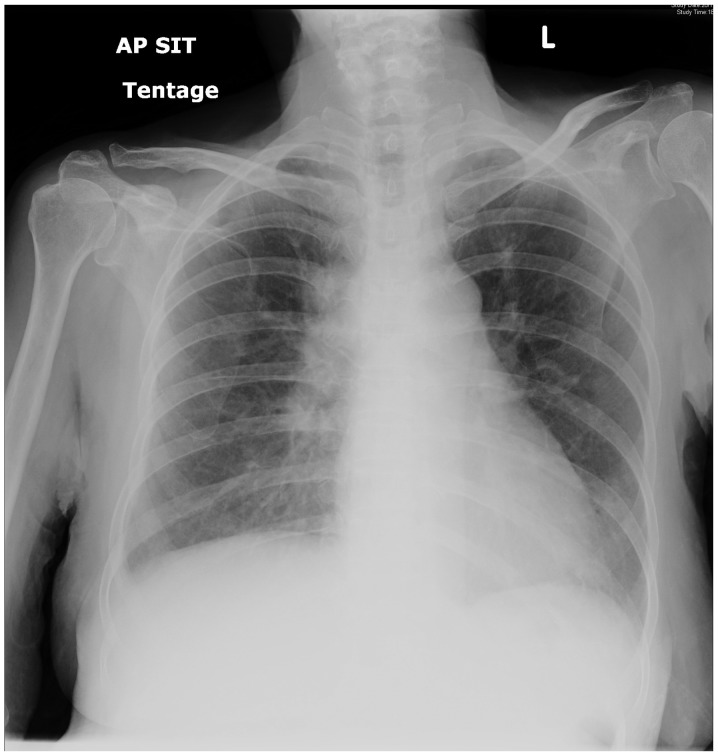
Chest X-ray posterior-anterior view showing no features that indicate a mass lesion.
